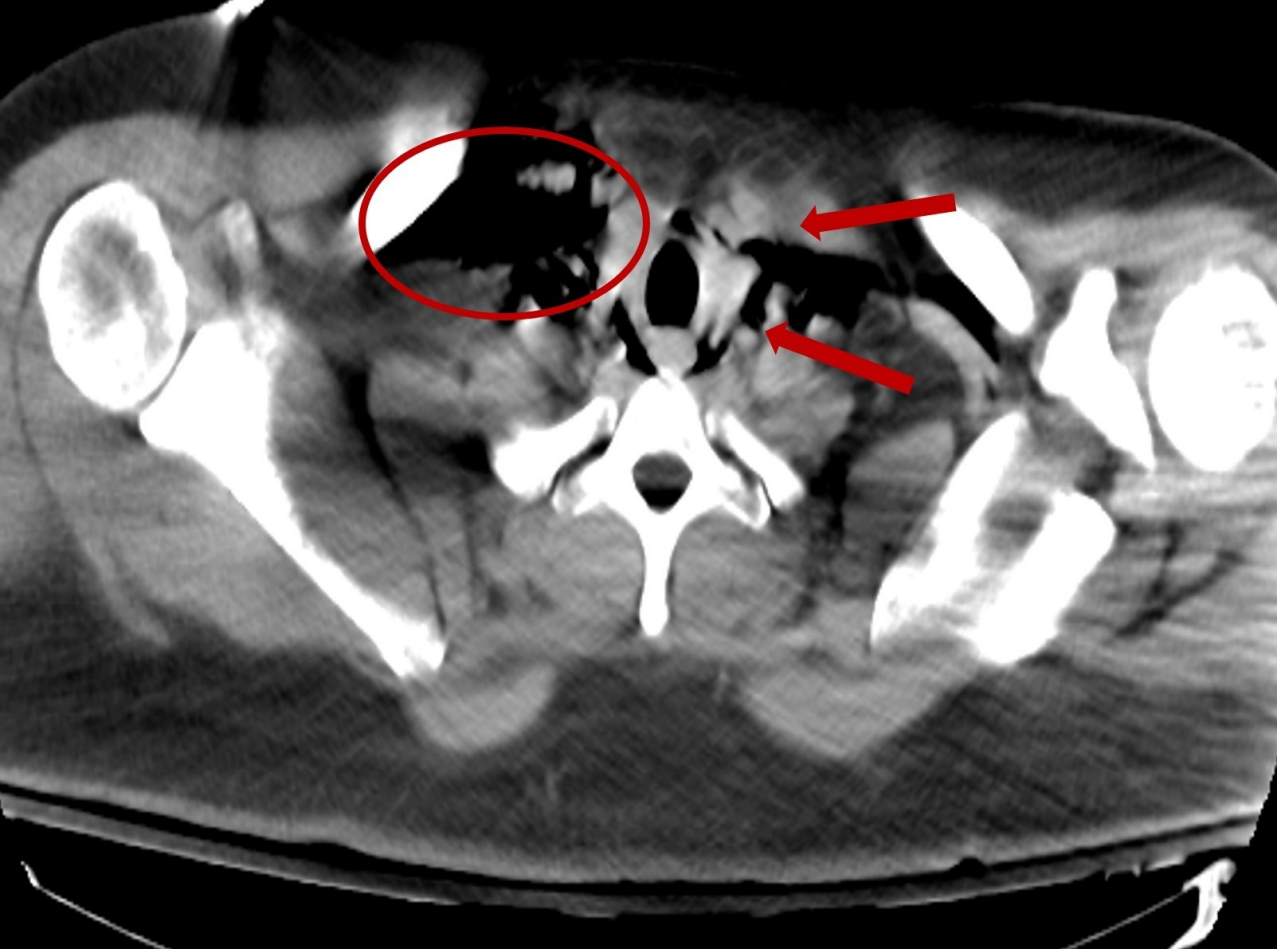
Computed tomography - axial view: extensive pneumomediastinum and emphysema Circle: emphysema; Arrows: pneumomediastinum.
